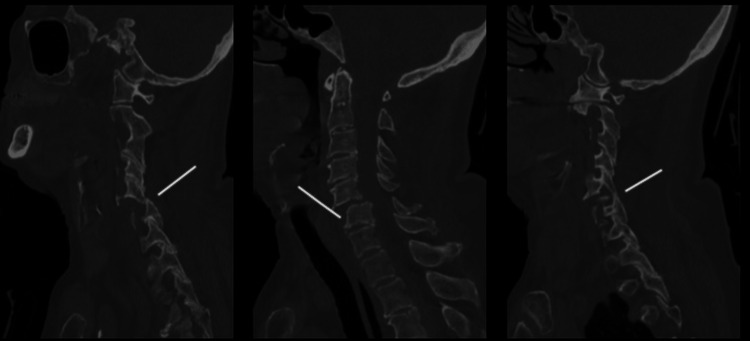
Sagittal CT of the cervical spine showing dislocation of the left facet joints at C5-C6 and perched right facet joint C5-C6, resulting in forward slip of C5 over C6. CT: computed tomography, C5/C6: cervical vertebrae 5/6.
